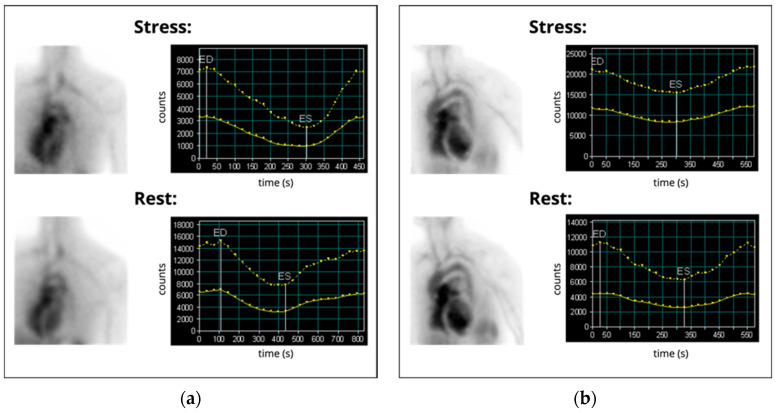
(a) Example of a physiological result in planar scintigraphy equilibrium radionuclide angiography: Normal LVEF and regular LVEF reaction to stress (rest: 56%, stress: 73%). (b) Example for a pathologic result: Decreased LFEF and inadequate LVEF reaction to stress (rest: 26%, stress: 24%). ED: end-diastolic, ES: end-systolic. Images were acquired at our institution.
